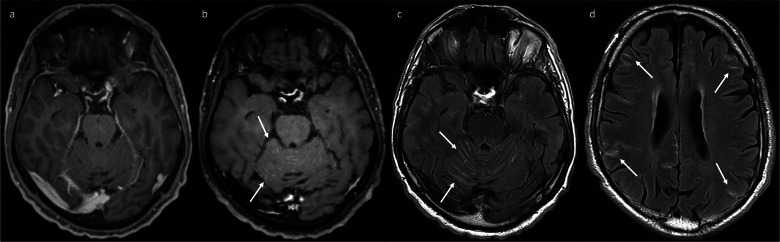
Different post-contrast imaging sequences in a patient with clinical features of irAE-encephalitis after cemiplimab for metastatic cutaneous squamous cell carcinoma, demonstrating the value of using different post-contrast sequences. Sulcal enhancement (arrows) visible on 3D-TSE (b) is barely visible on 3D-GRE (a). It is arguably best appreciated on the post-contrast FLAIR sequence (c), which also demonstrated enhancement within several cerebral sulci further superiorly (d)
